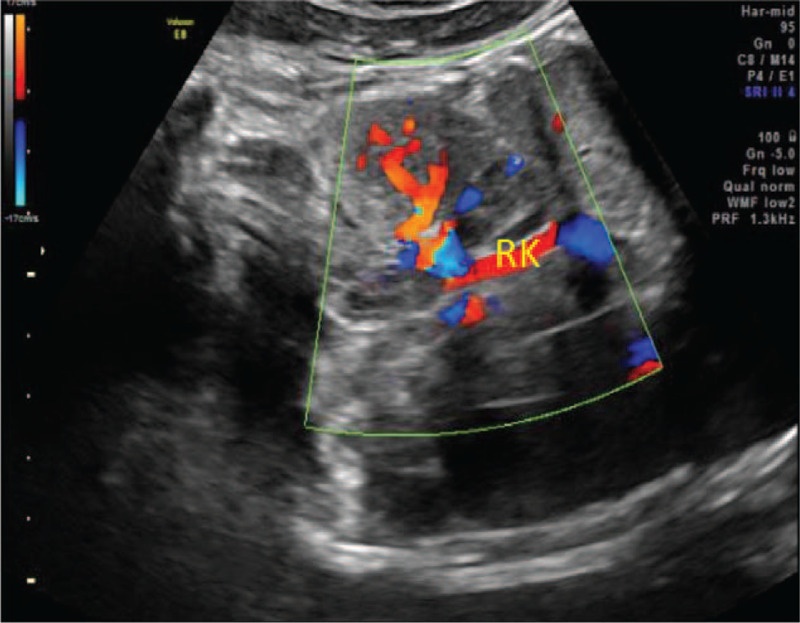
Color Doppler ultrasound shows a large nutrient vessel entering the inside of the tumor.
